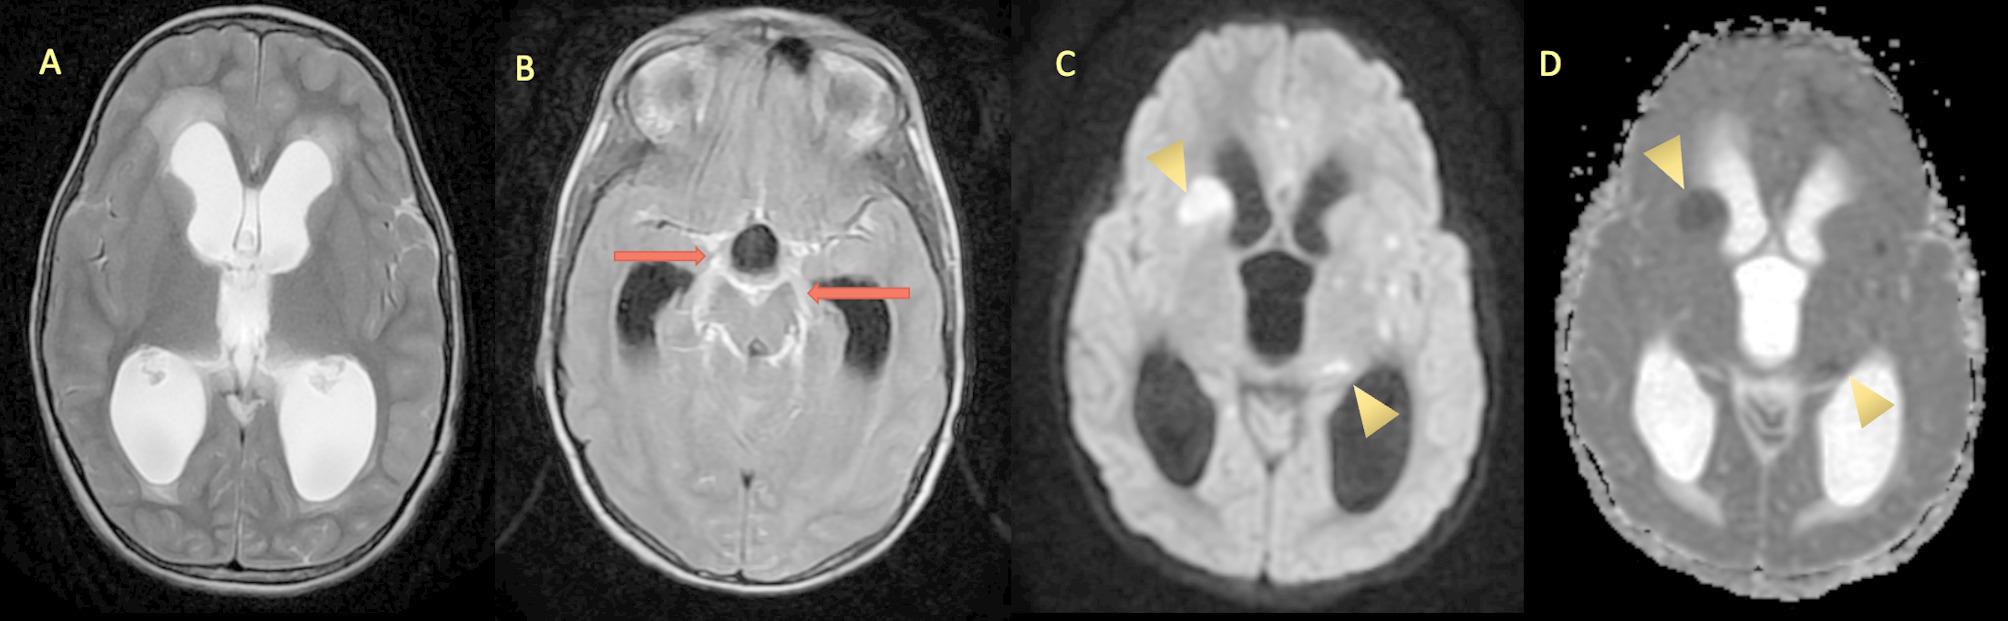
MRI brain. Axial T2W image (A) showing moderate hydrocephalus, post contrast T2 FLAIR axial image (B) demonstrating basal exudates with abnormal enhancement along the perimesencephalic and suprasellar cisterns (red arrow). Axial DW & ADC images (C & D) showing restriction of diffusion in the right caudate nucleus, and bilateral thalamostriate regions (yellow arrow heads)
